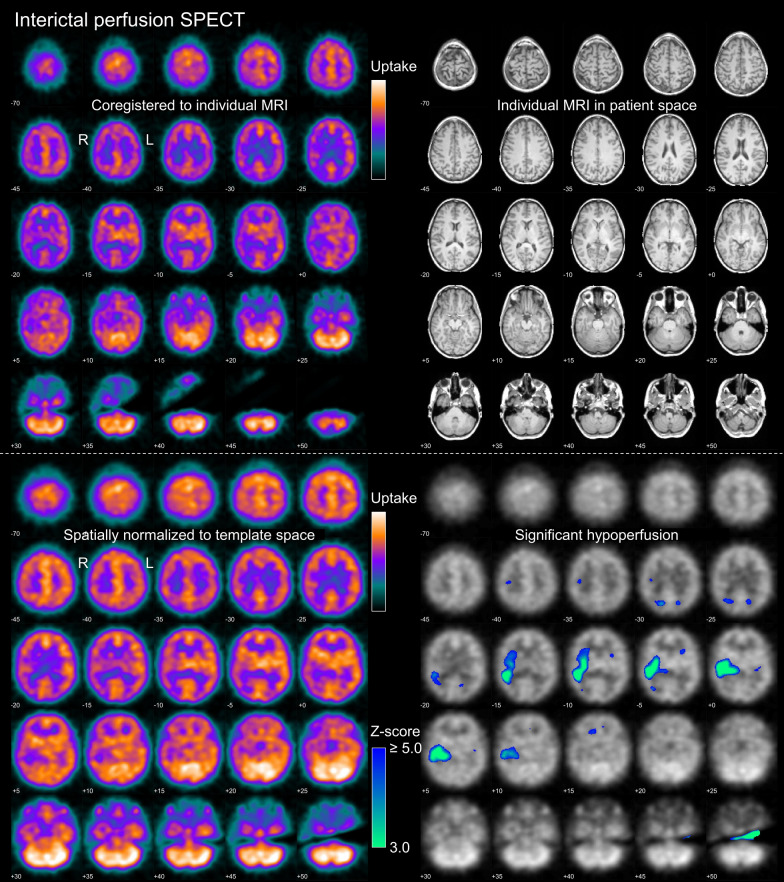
Standardized display for visual reading in the “interictal only” setting. The upper and lower parts were provided as page 1 and page 2 of a 2-page pdf-document. On the first page, the left side shows the patient’s interictal perfusion SPECT coregistered to the individual T1-weighted MRI shown on the right. On the second page, the left side shows the patient’s interictal perfusion SPECT after spatial normalization to MNI space, the right side shows the statistical hypoperfusion map thresholded at z ≥ 3.0 and overlaid to the patient’s interictal SPECT. The upper threshold of the color bar for the display of the SPECT images was set to the maximum of the 3-dimensional SPECT image volume, separately for each SPECT scan. The lower threshold of the color bar was set to zero in all cases. The example images were acquired with ^99m^Tc-HMPAO in a 28y old woman with normal MRI
